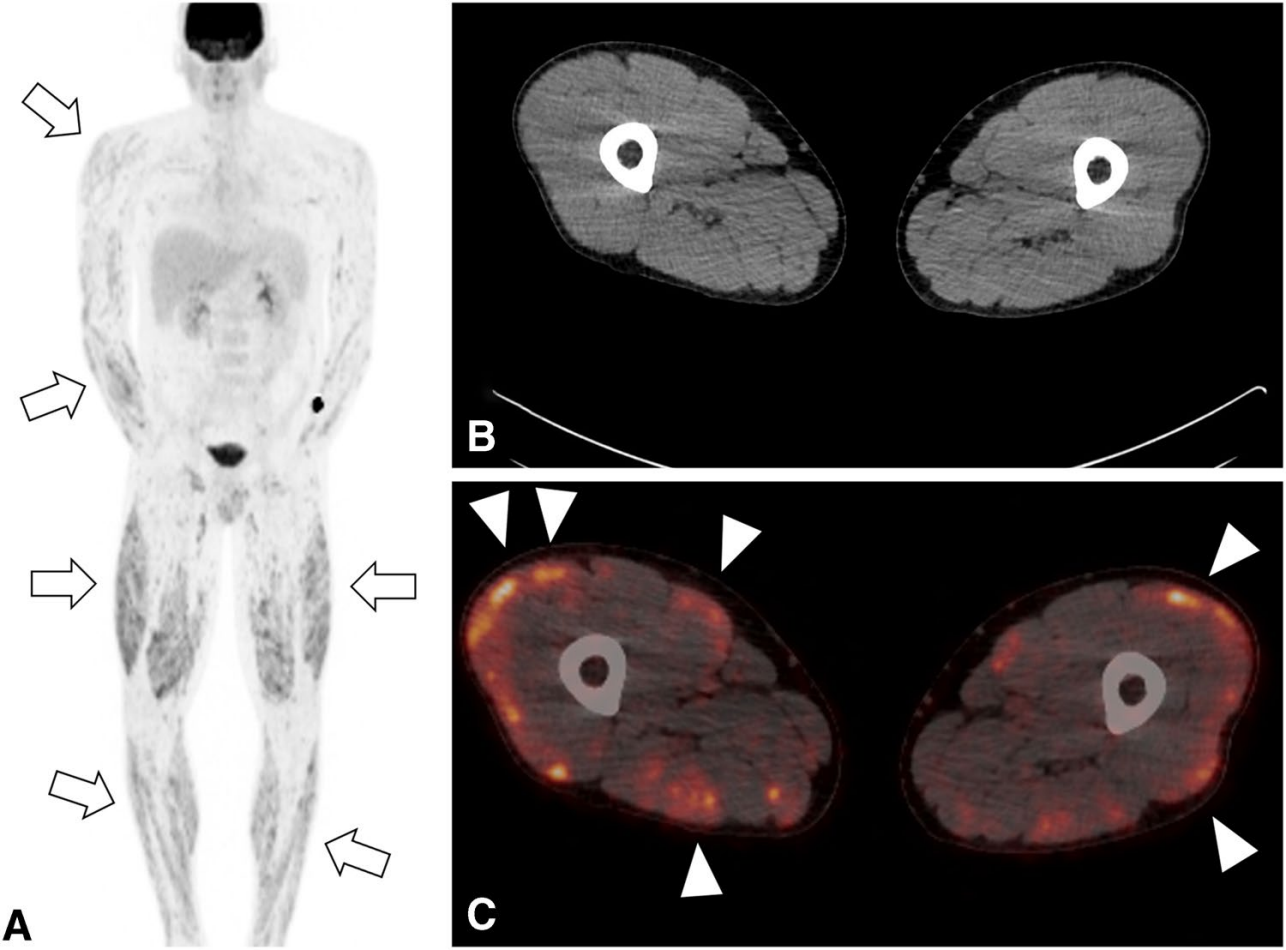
Coronal maximum intensity projection (MIP) of the 18-F-fluorodeoxyglucose positron emission computed tomography (PET/CT), A displaying increased metabolic activity of the muscles of the upper and lower extremities (arrows). Axial CT (B) and fused PET/CT (C) images of the thigh with nodular appearance of metabolic activity of the muscles (arrowheads) without a morphological correlate in the CT
